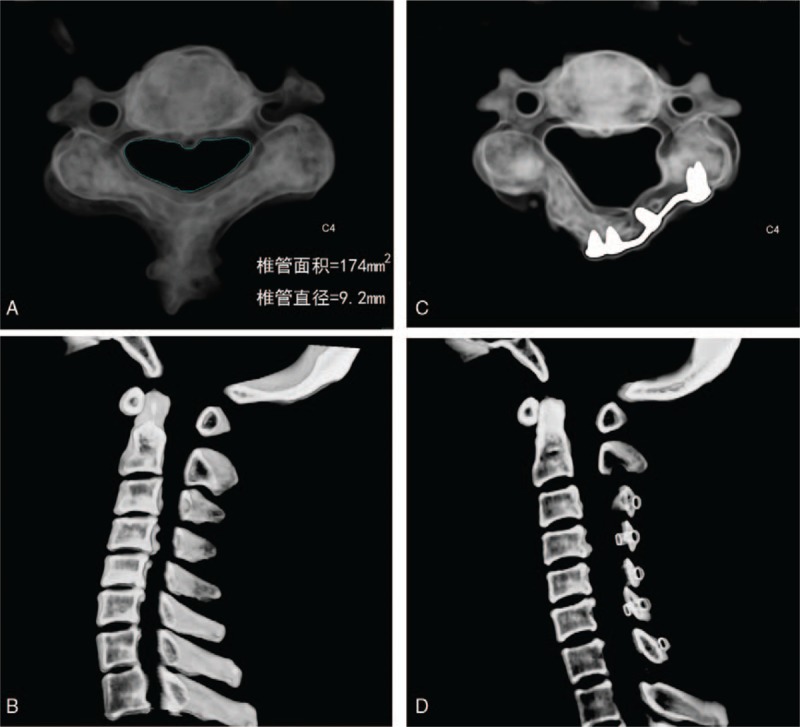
The CT scanning images before and after operation. (A) and (B) are the CT scanning images before the surgery operation; (C) and (D) are after the surgery.
